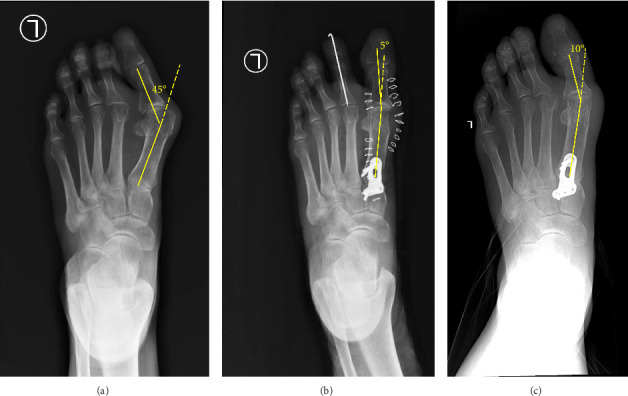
Dorsoplantar foot radiograph showing hallux valgus angle (HVA) measurements: (a) preoperative; (b) immediate postoperative; (c) 6-months postoperative.
